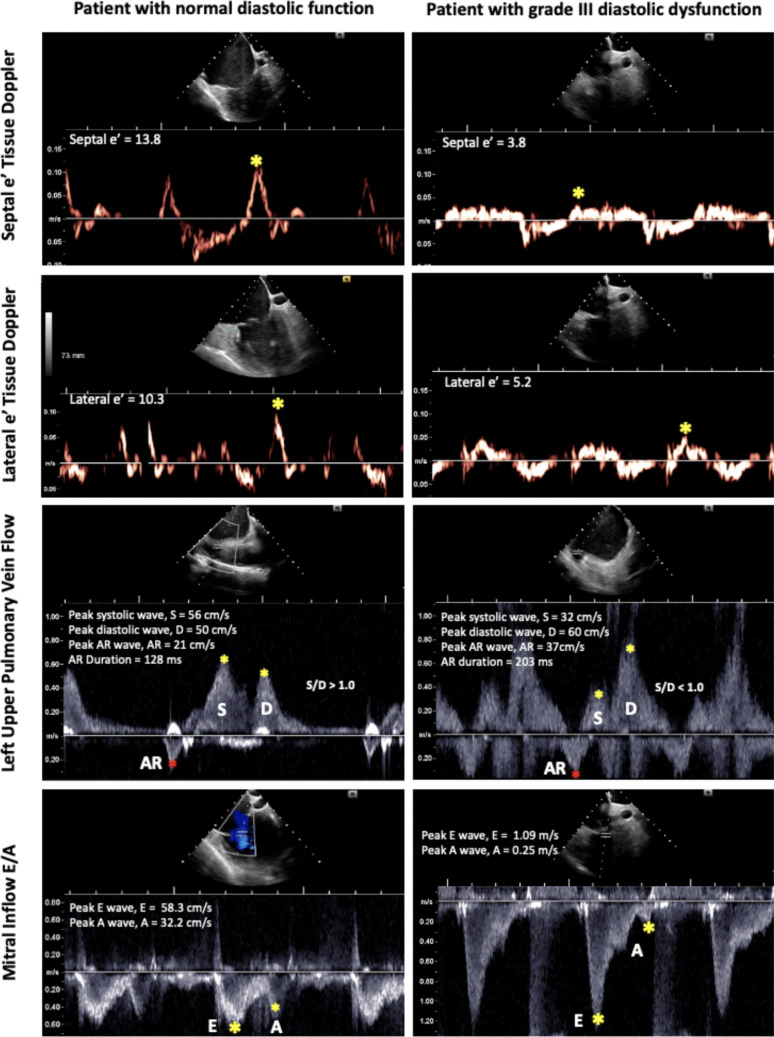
Intracardiac echocardiographic interrogation of left ventricular diastolic function in patients during AF ablation. A, peak mitral inflow velocity in late diastole; AR, atrial reversal; D, diastolic wave; e′, early diastolic mitral annular velocity using tissue Doppler imaging; E, peak mitral inflow velocity in early diastole; S, systolic wave
